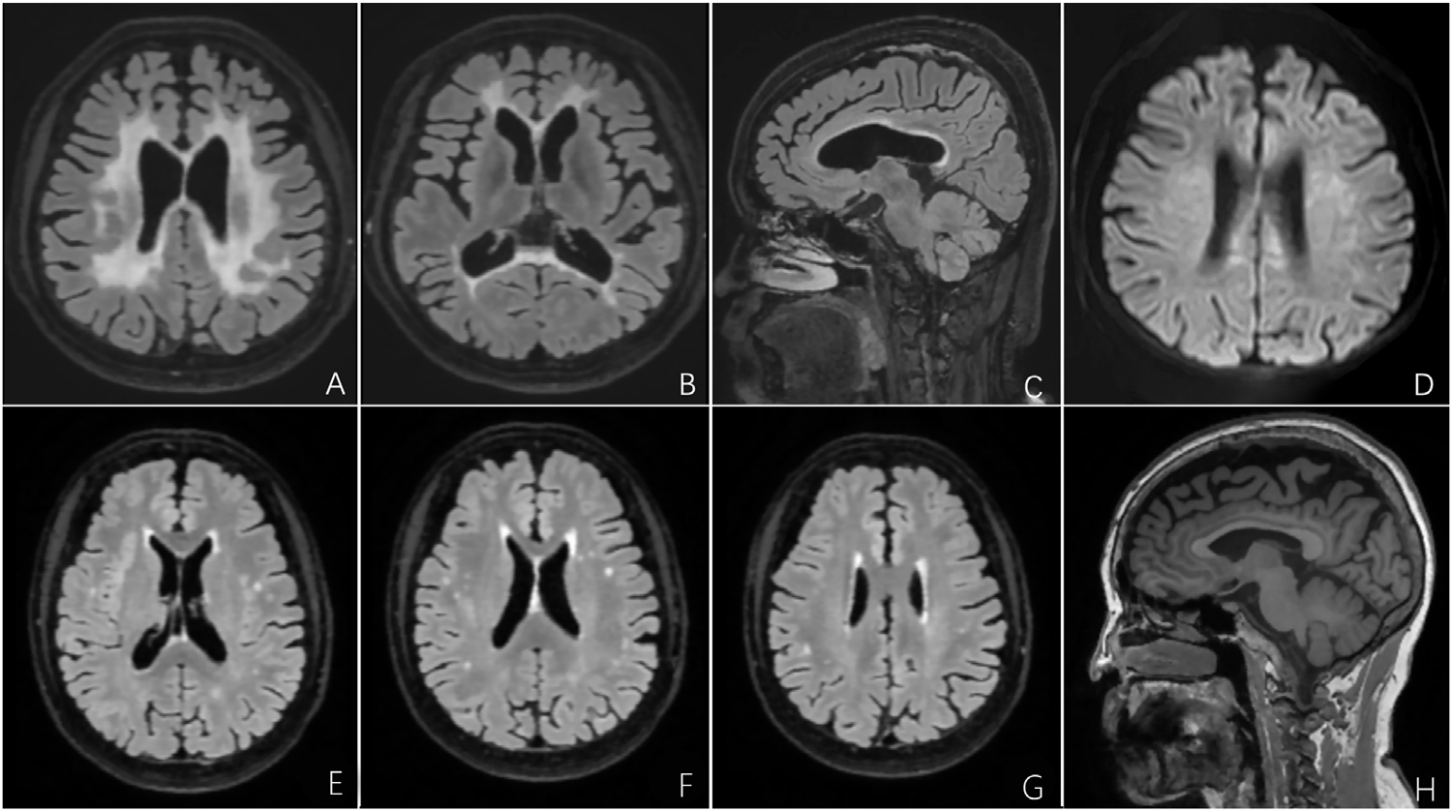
Brain MRI of the proband and her mother with CSF1R-microglial encephalopathy in the second family. Bilateral confluent and diffuse deep white matter hyperintensities involving periventricular areas, corona radiate and the corpus callosum on the FLAIR imaging (A,B) and DWI (D). Sagittal brain FLAIR shows abnormal hyperintensities and thinning of the corpus callosum, and extensive cortical atrophy (C). Bilateral confluent deep white matter hyperintensities involving periventricular areas, corona radiate and the trunk of corpus callosum on the FLAIR imaging (E–G). Sagittal brain T1-weighted imaging shows significant thinning of the corpus callosum, and extensive cortical atrophy (H).
